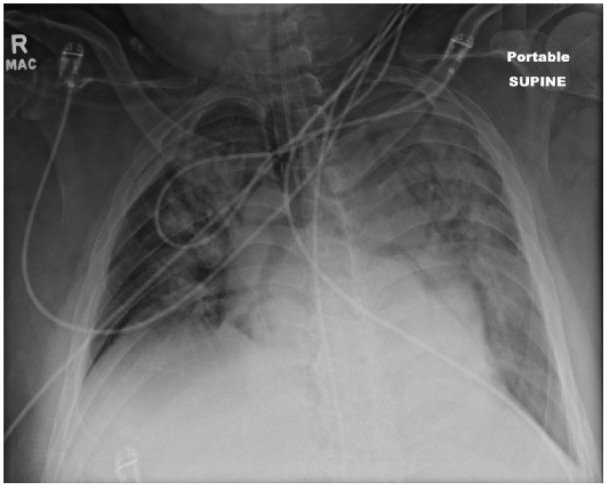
AP view of patient’s chest radiograph showing bilateral airspace consolidation and cardiomegaly suggestive of pulmonary edema.
